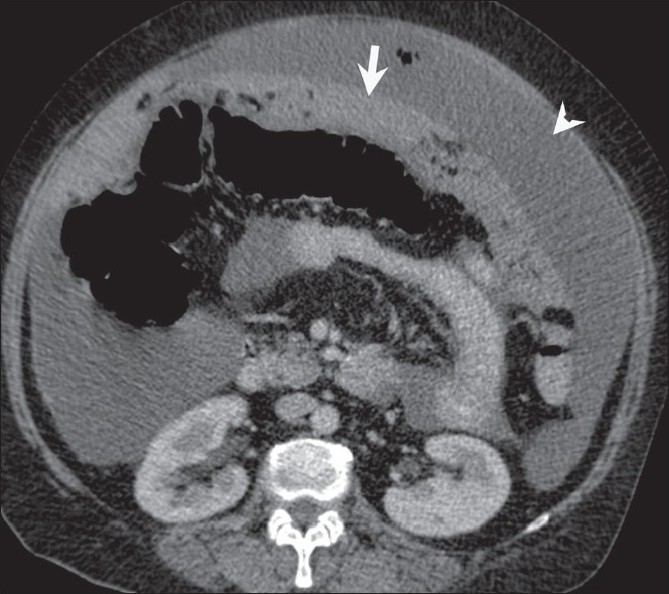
Peritoneal carcinomatosis. Omental caking (arrow) and ascites (arrowhead) are seen on an axial, contrast-enhanced CT scan of the abdomen, in a 55-year-old woman, a known case of carcinoma ovary with raised CA-125 levels
